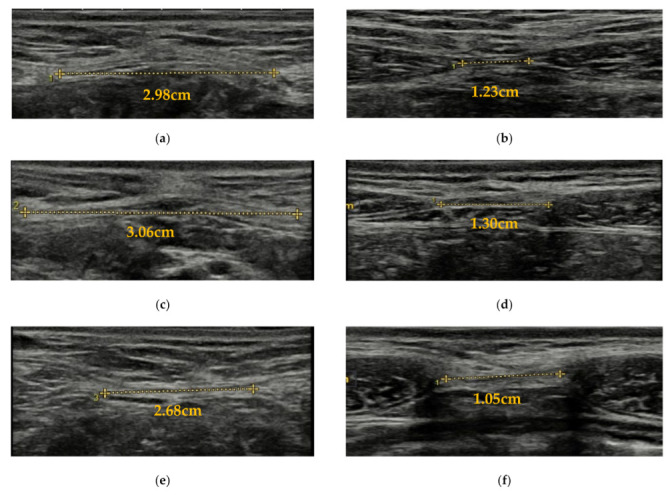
IRD measurement through ultrasound imaging assessment in the different conditions and locations. (a) IRD measurement at 2 cm above the umbilicus at rest; (b) IRD measurement at 2 cm below the umbilicus at rest; (c) IRD measurement at 2 cm above the umbilicus during ADIM; (d) IRD measurement at 2 cm below the umbilicus during ADIM; (e) IRD measurement at 2 cm above the umbilicus during curl-up; (f) IRD measurement at 2 cm below the umbilicus during curl-up. Abbreviations: IRD, inter-rectus distance; ADIM, abdominal draw-in maneuver.
